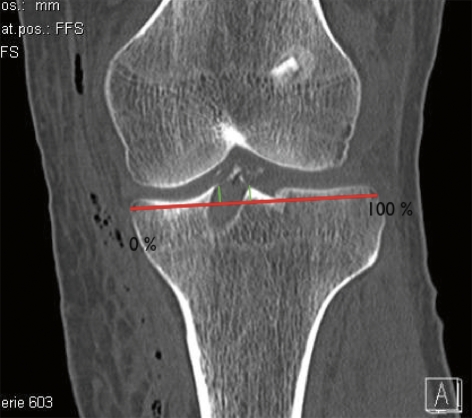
Medial-lateral measurement in the coronal CT reconstruction of the tibial tunnel. The tunnel measurement is compared to the line through the most medial and lateral part of the tibial plateau.
